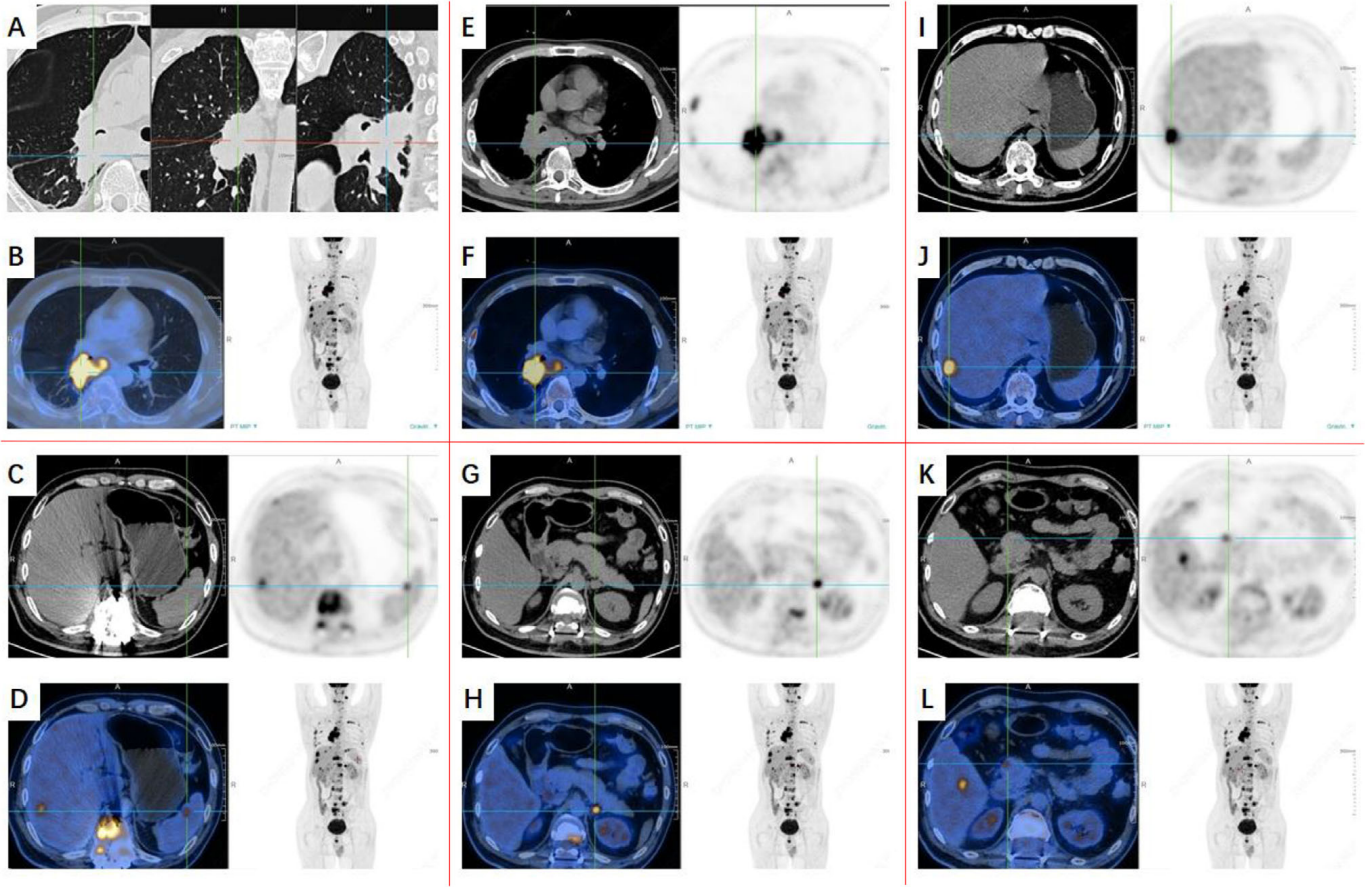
Imaging phenomes of positron emission tomography/computed tomography (PET/CT) 4 months after onset (continuation of Figure 1): Lung MT with multiple systemic metastases, more bone metastases and liver metastases, brain metastasis, left submaxillary lymph node metastasis, spleen and left adrenal gland metastasis. A,B,E,F, PET/CT images of the chest. C, D, and G‐L, PET/CT images of abdomen
